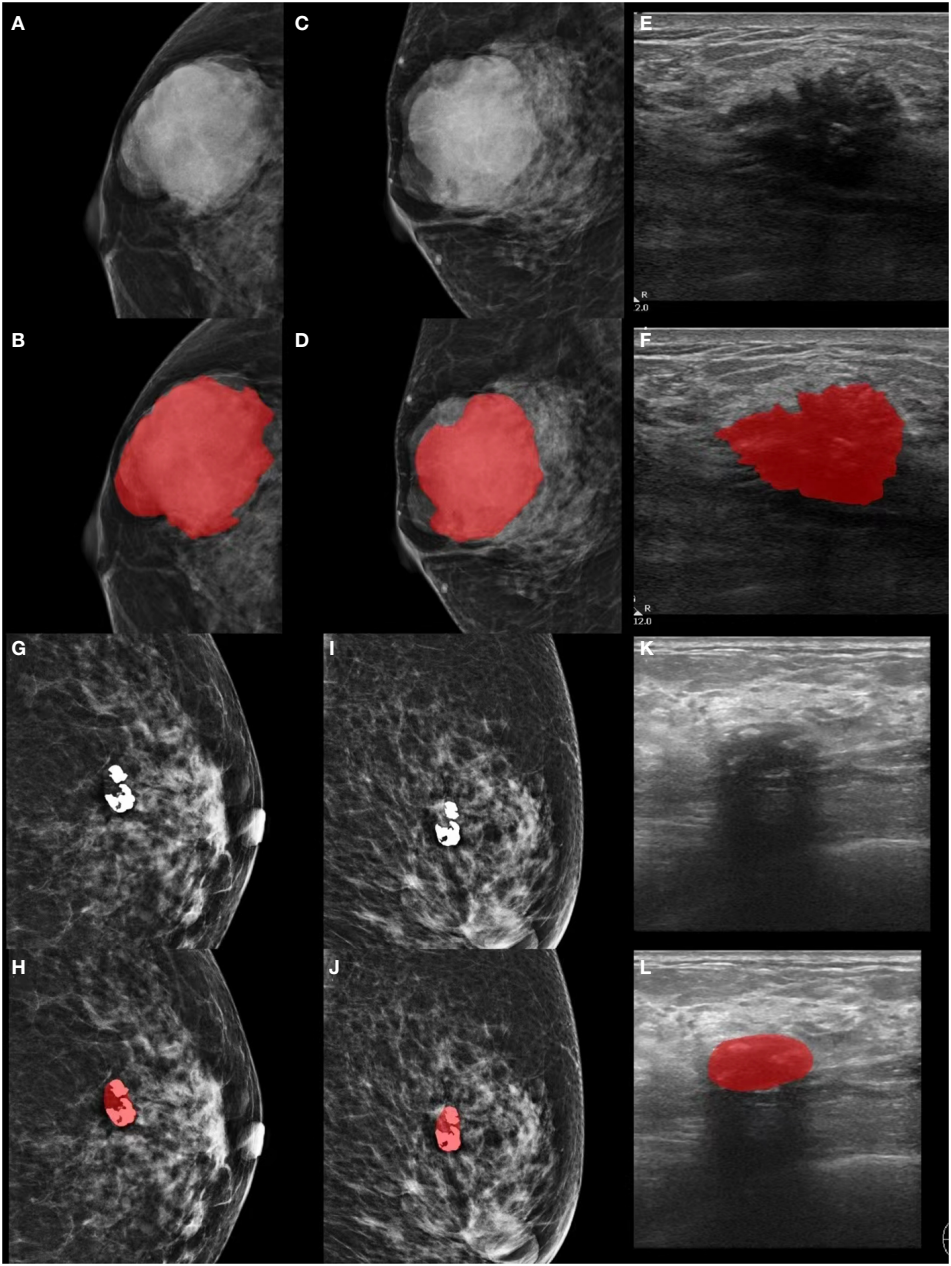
Lesion segmentation in MG and GSUS images. (A-F) Displaying the original MG, GSUS images, and segmentation of a 32-year-old female patient with confirmed breast adenosis. (G-L) Presenting the original MG, GSUS images, and segmentation of a 57-year-old female patient with confirmed IDC. MG, mammography; GSUS, gray-scale ultrasound; IDC, invasive ductal carcinoma.
